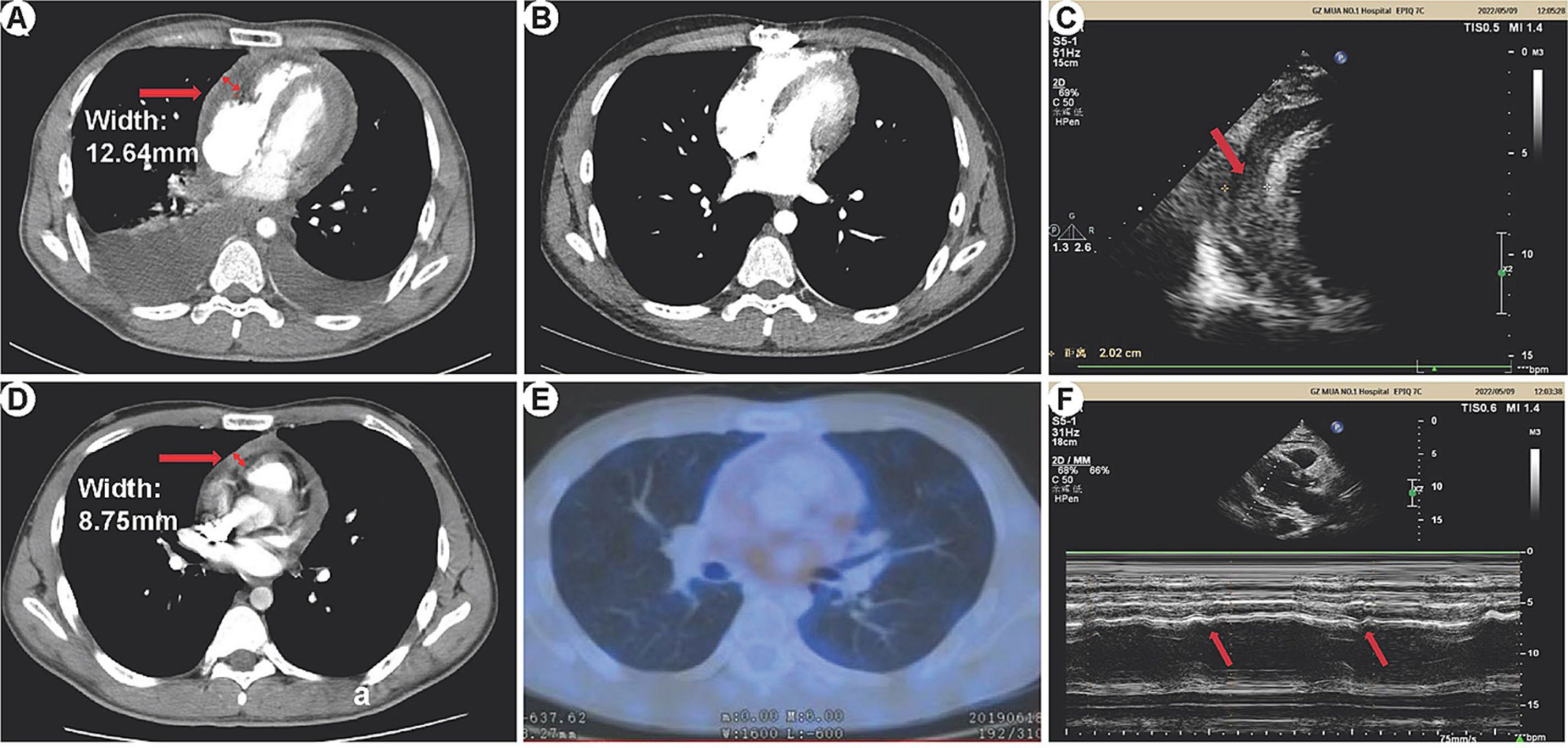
HRCT, 18F-FDG PET/CT, and echocardiography manifestations of constrictive pericarditis. (A) Preoperative CT: Bilateral pleural effusion is visible, more prominent on the right side. The pericardium appears significantly thickened, measuring 12.64 mm at its thickest part. (B) Postoperative CT: No pleural effusion is observed in either chest cavity. There is no evidence of pericardial thickening or pericardial effusion. (C) Echocardiography: The ventricular septum exhibits bouncing motion (indicated by arrows), characterized by a short backward motion in early diastole. (D,E) 18F-FDG PET/CT: Pericardial thickening is observed with unevenly increased glucose metabolism. The maximum thickness of the pericardium measures 8.75 mm. (F) Echocardiography: The pericardium displays inhomogeneous high-weak echogenic changes, indicative of pericardial thickening (arrows).
